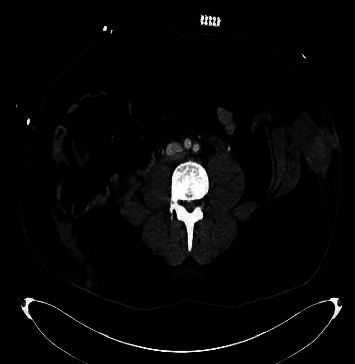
Abdominal CT. Transection of the lateral abdominal wall muscles along with herniation of bowel contents.
